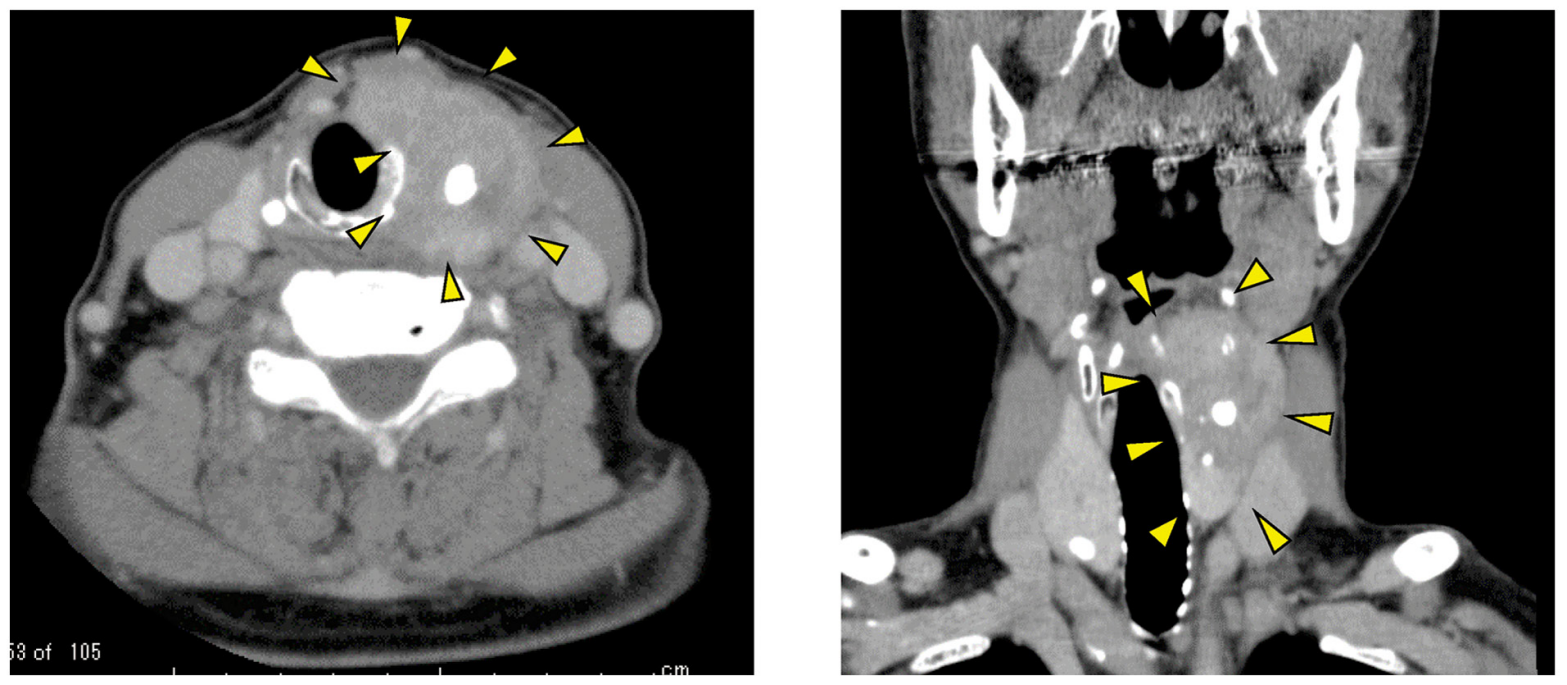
Pre-operative computed tomography images revealed a calcified thyroid tumour (surrounded by arrowheads). The tumour was invasive and extended from the left side of the thyroid cartilage to the upper part of the cricoid cartilage.
